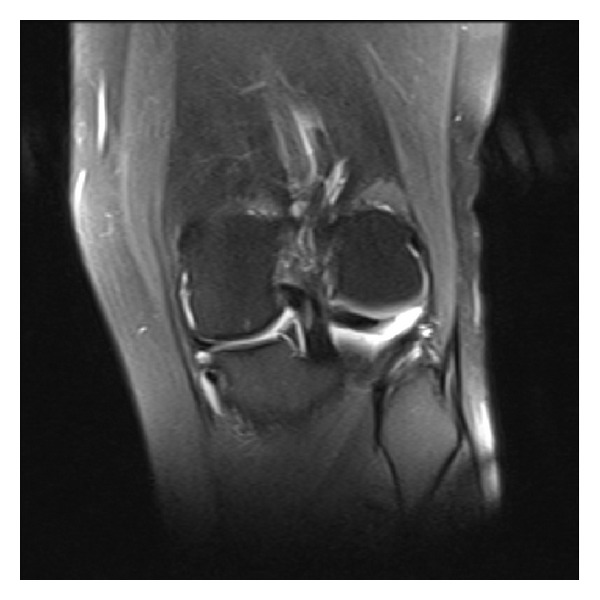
Coronal MR image demonstrates decreased volume of the medial meniscus and a meniscal fragment within the intercondylar notch.
